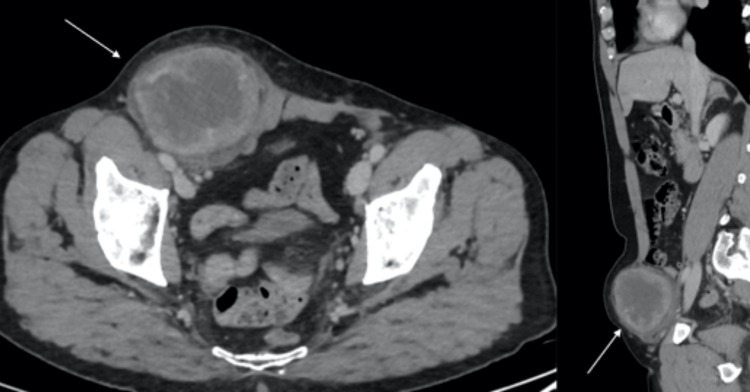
Axial and sagittal contrast-enhanced computed tomography scan of the abdomen and pelvis showing a right inguinal lesion (white arrows)
